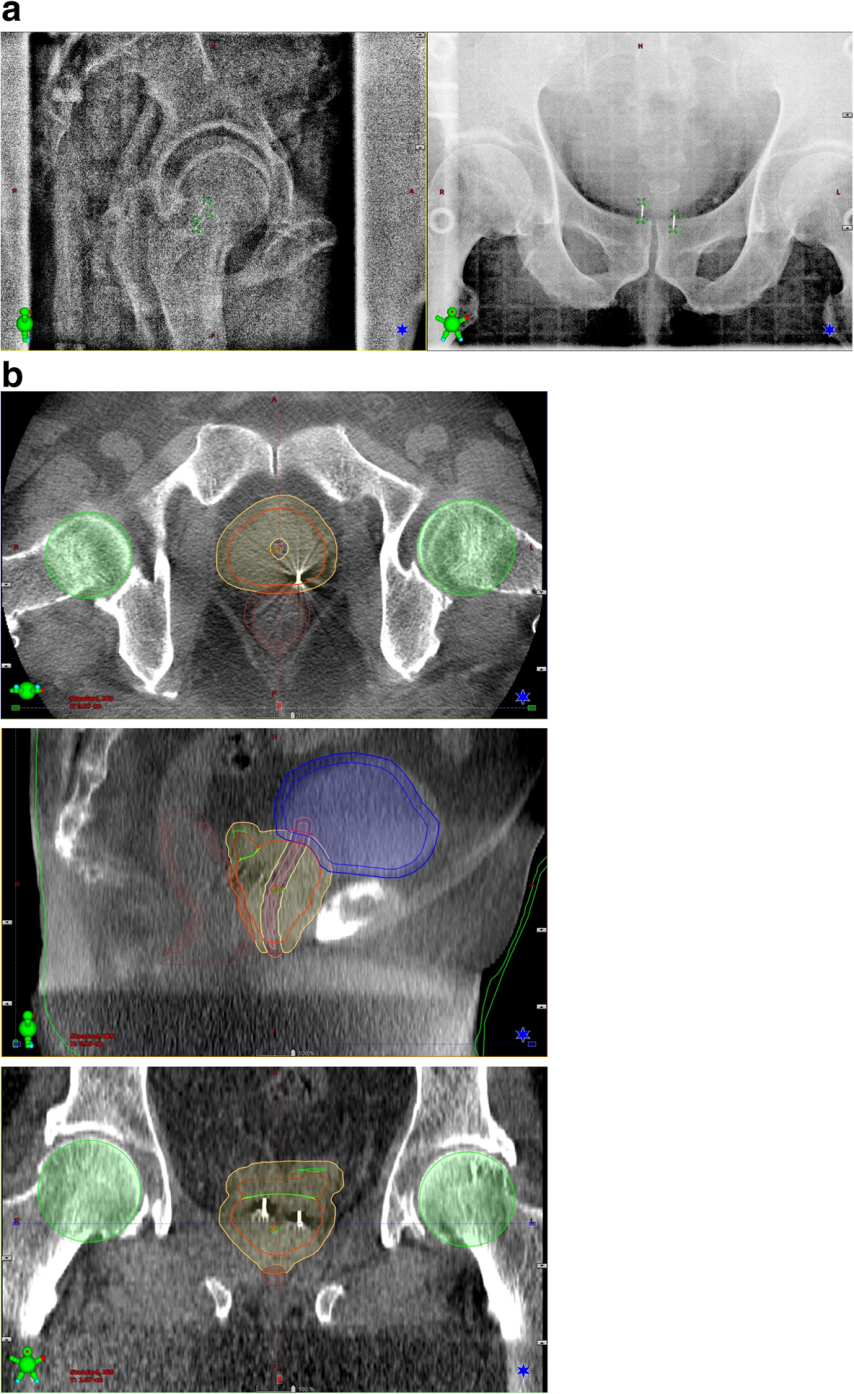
Image datasets of kV fiducial on-board imaging (a) and kV cone-beam computed tomography (b) with the contours used for matching
